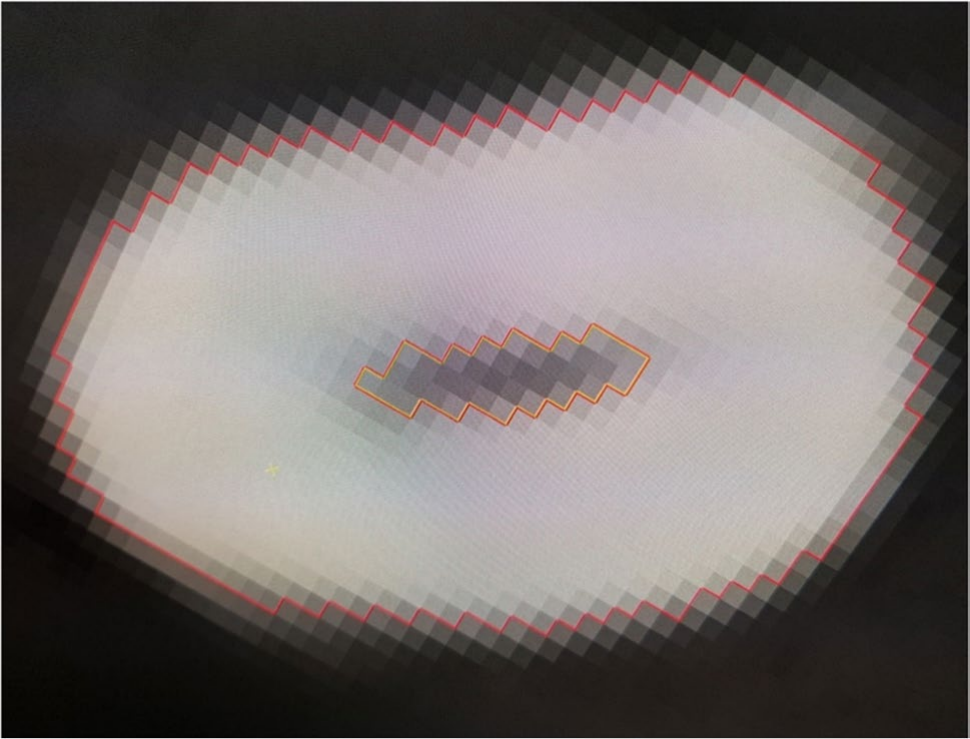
Tooth 43, CBCT dataset, enlarged transversal view. Manual segmentation. Red line, external tooth boundary. Orange line, segmentation of the pulp cavity. Even with semi-automatic segmentation, the examiner must first determine the gray values that are to be considered as pulp by the software
